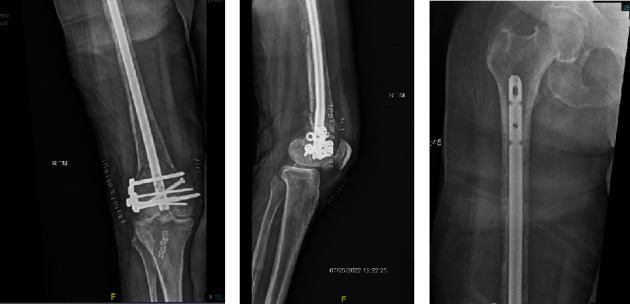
Postoperative radiographs of the femur showing properly placed retrograde femoral nailing system.
